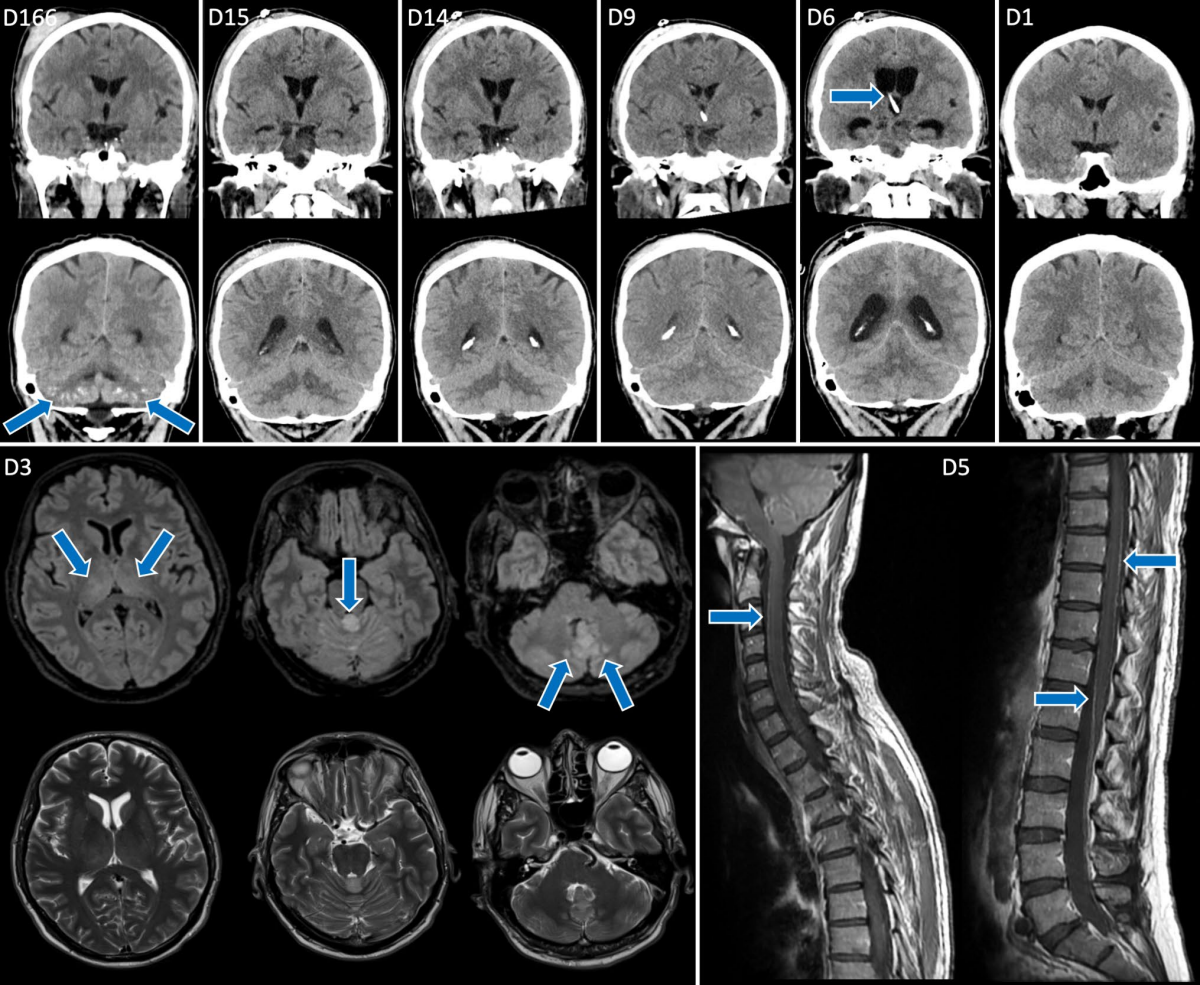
Neuroimaging demonstrating TBE meningoencephalitis. At admission day 1 (D1), brain computed tomography (CT) was normal. Brain magnetic resonance imaging (MRI) on day 3 (lower left panel) revealed edema (arrows) in the thalami bilaterally, superior vermis and medially in the cerebellum. Spinal MRI on day 5 (lower right panel) revealed leptomeningeal enhancement (arrows). On day 6, obstructive hydrocephalus occurred, and the patient was treated with ventricular drainage (arrow), which reconciled the increased pressure (D9), and the drainage could subsequently be removed without hydrocephalus (D14, D15). At a brain CT scan 5.5 months later due to trauma (acute right frontoparietal extracranial hematoma present), extensive brain calcifications in the cerebellar parenchyma had developed (arrows)
